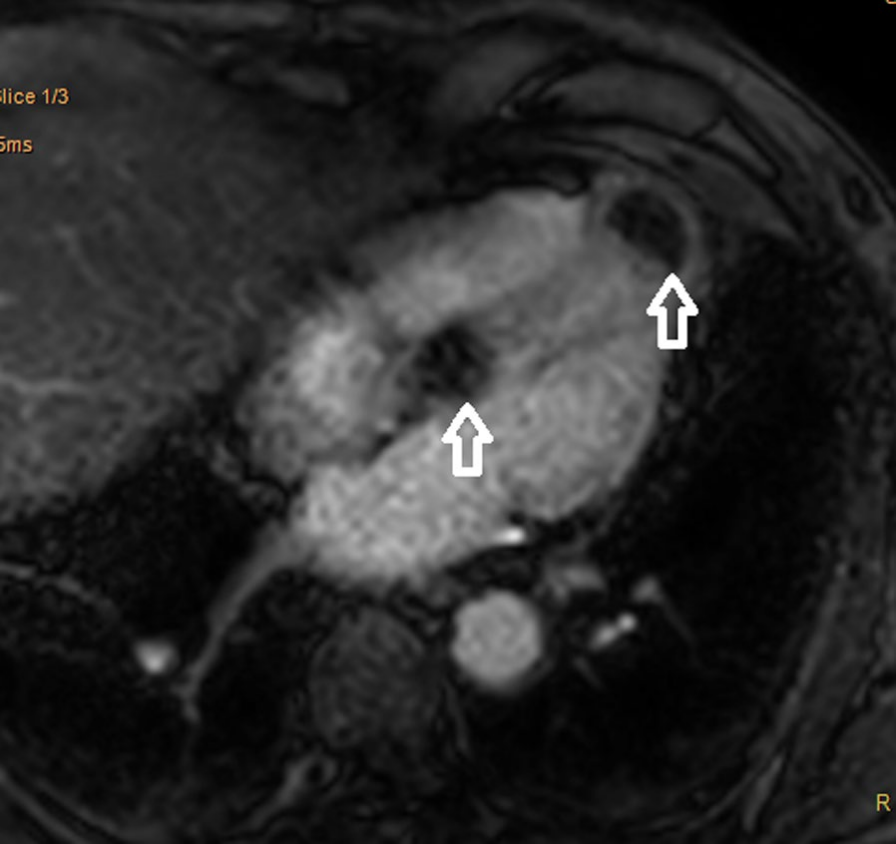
Cardiac Magnetic Resonance Imaging: First pass perfusion in 4-chamber view demonstrating hypoperfusion of the lesions (indicated by arrows) when compared to normal myocardium
